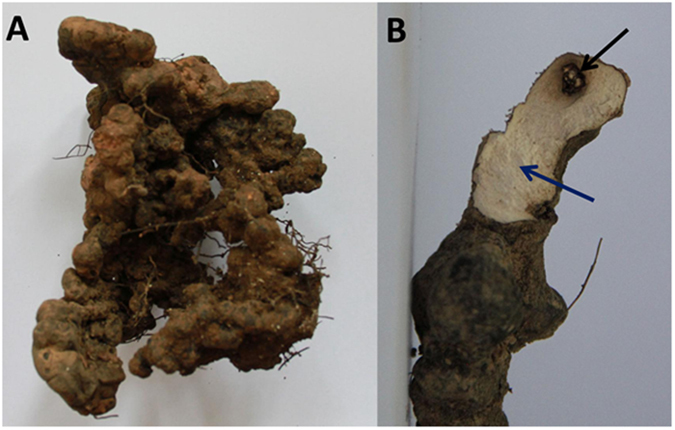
(A) The sclerotium of *Polyporus umbellatus* collected from Guxian, Shanxi. The surface of the sclerotium was attached to and penetrated by *A. mellea* rhizomorphs. (B) The internal part of the sclerotium. The black region (CT) surrounding the area invaded by rhizomorphs of *A. mellea* (black arrow) and and the none-invading region (CK) was white in color (blue arrow).
